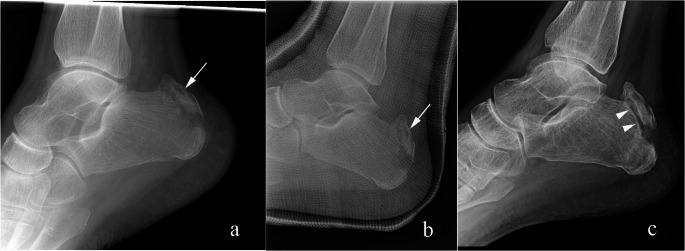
Type I calcaneal tuberosity avulsion fracture. 71-year-old woman with pain after a fall. Lateral radiographs show a calcaneal tuberosity fracture (arrows in a and b) at presentation (a) and at 6 weeks follow up (b). Due to comorbidities the patient was treated conservatively. At 3 months follow up (c), there is non-union of the fracture fragment (arrowheads) with no osseous bridging and sclerotic margins
